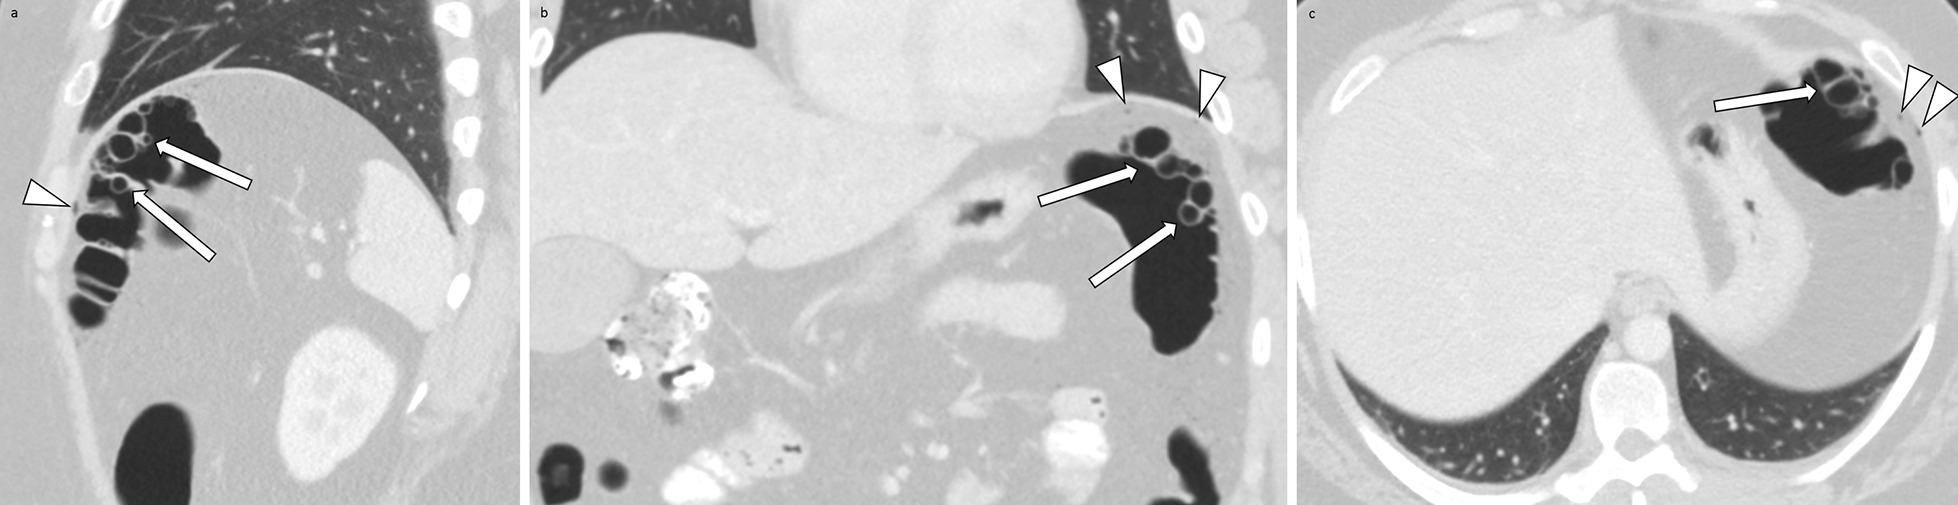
Computed tomography scan of the abdomen/pelvis sagittal view (a), coronal view (b), and axial view (c) demonstrating findings of numerous cysts within the wall of the splenic flexure of the colon (arrows) with trace pneumoperitoneum in the subphrenic spaces (arrowheads)
